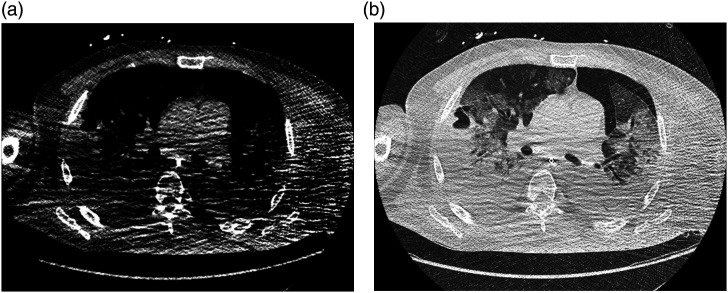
49-year-old male patient, who presented with progressive dyspnea and fever. (a) Chest CT with lowered arms and major artifacts throughout the entire thorax resulting in a quality rating of 3 (non-diagnostic). (b) Lung window at the same position shows minor artifacts in the lung parenchyma. DLP: 45.9 mGy*cm.
